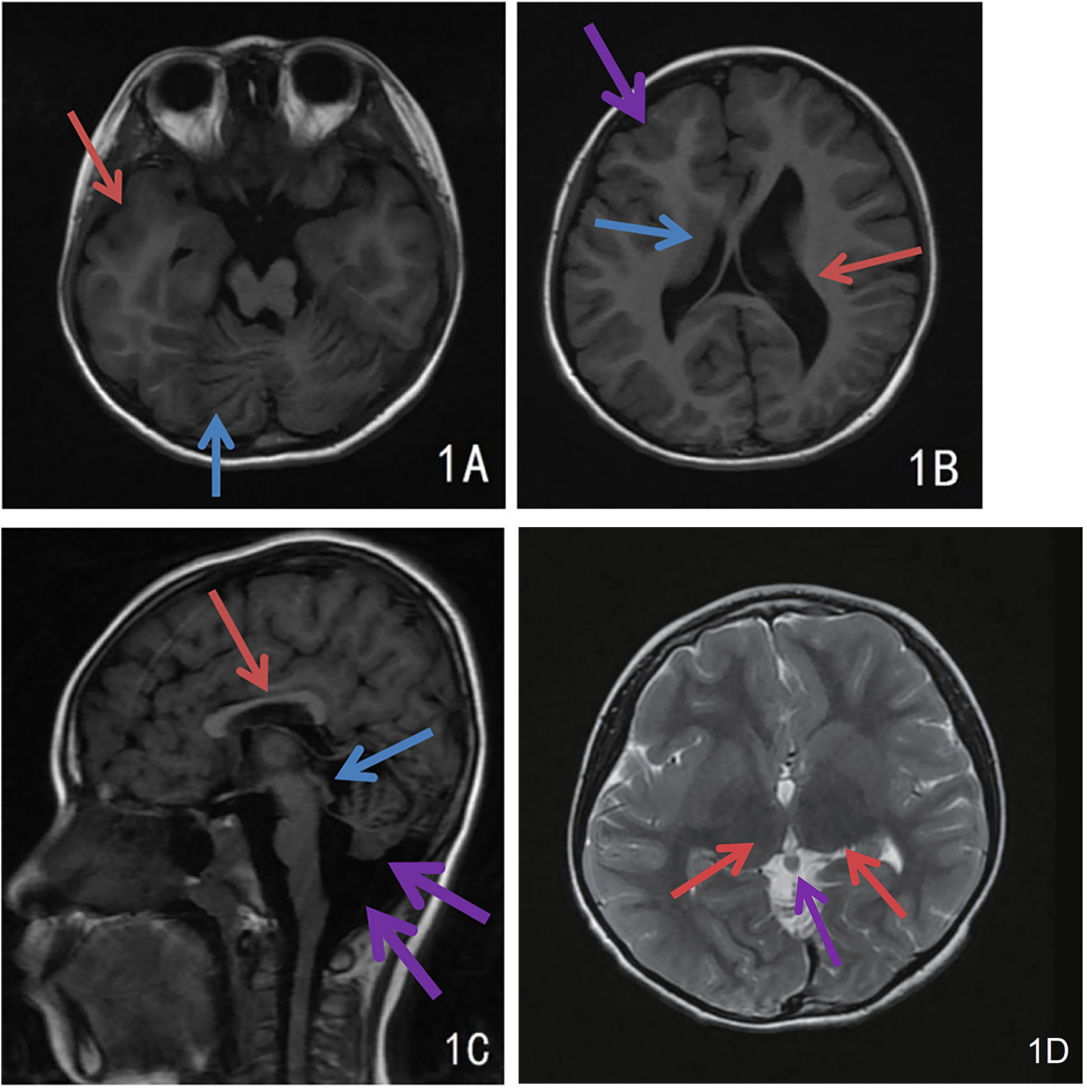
Cranial MRI findings of the child. (A) Extensive and deep cerebellar sulcus in the cerebellar hemispheres (blue arrow), cortical thickening and dysplasia of the right temporal lobe (red arrow); (B) Uneven cortical hypertrophy, midline deviation, dilated lateral ventricles and hypoplasia of the right frontal lobe, irregular cortical morphology of right frontal lobe with dysplasia (purple arrow), hypertrophic caudate nucleus at right ventricular border (blue arrow), dilatation of left ventricle (red arrow); (C) Irregular cortical hypertrophy, short corpus callosum (red arrow), hypoplasia of the cerebellar earthworms with widened retrocerebellar CSF space (purple arrow), and heterogeneous signal intensity in pineal region (blue arrow); (D) Bilateral thalamic hypertrophy (red arrows), nodular shadow in the pineal region (purple arrow), possibly a pineal cyst.
